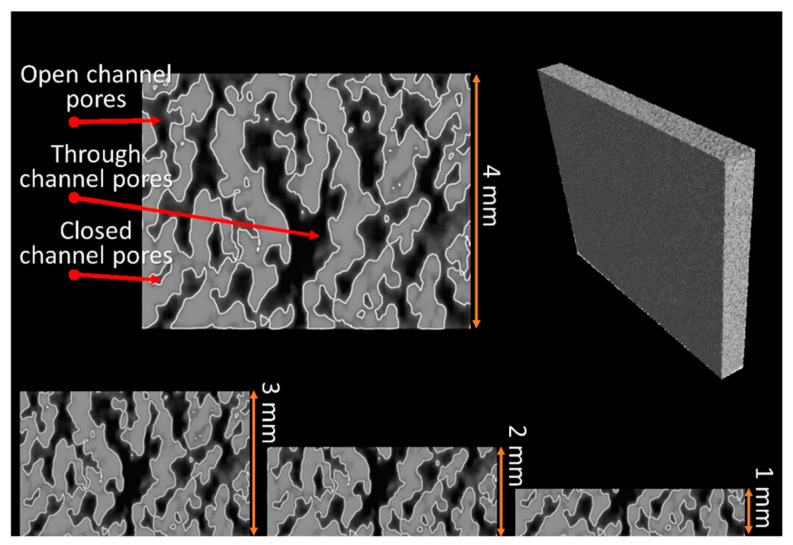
CT images of pores changing with thickness of samples. Vertical direction corresponds to the thickness direction of the samples. The inset of 1~3 mm represents the thickness of the samples.
